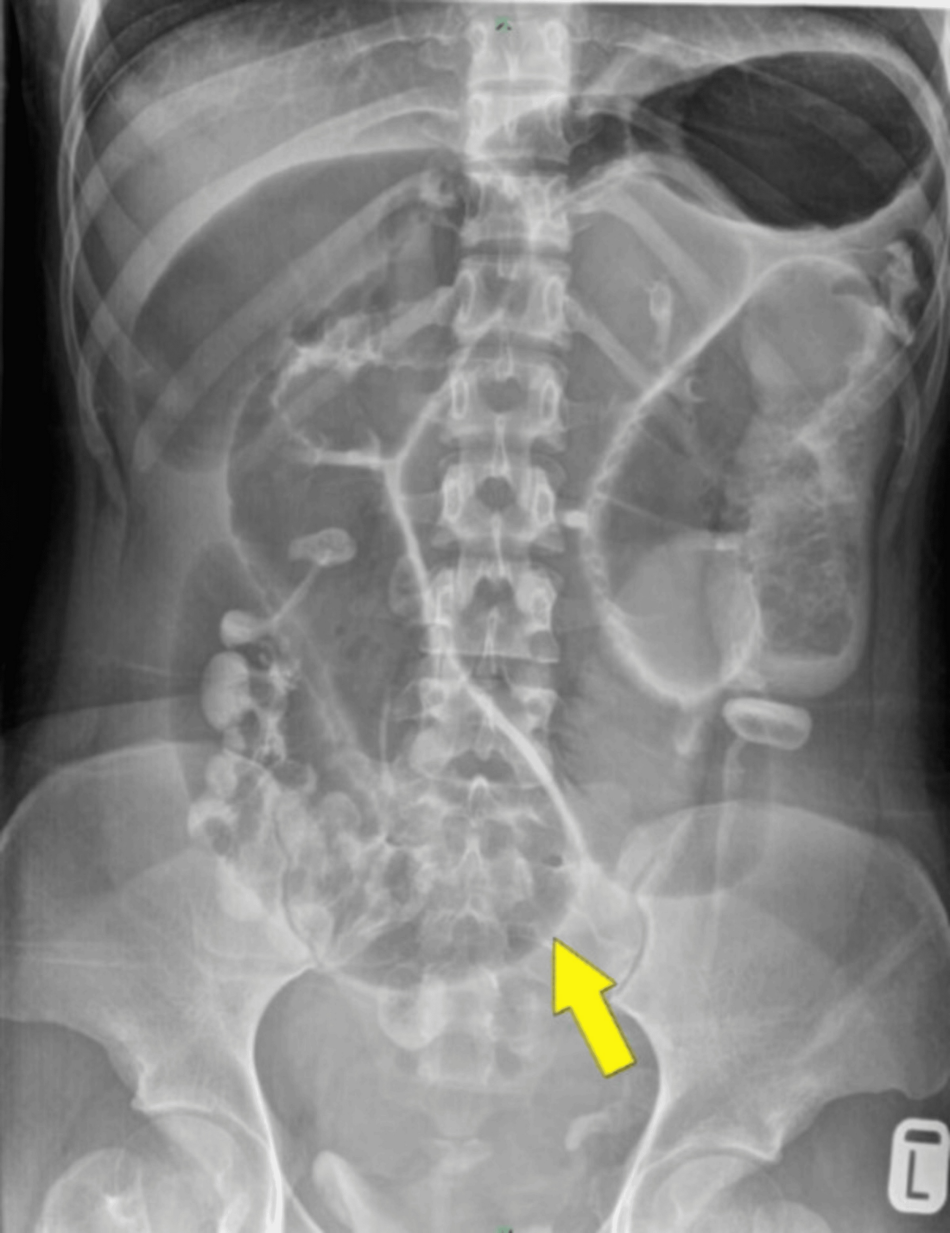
Plain abdominal X-ray in supine position showing grossly dilated small bowel loops (yellow arrow).
